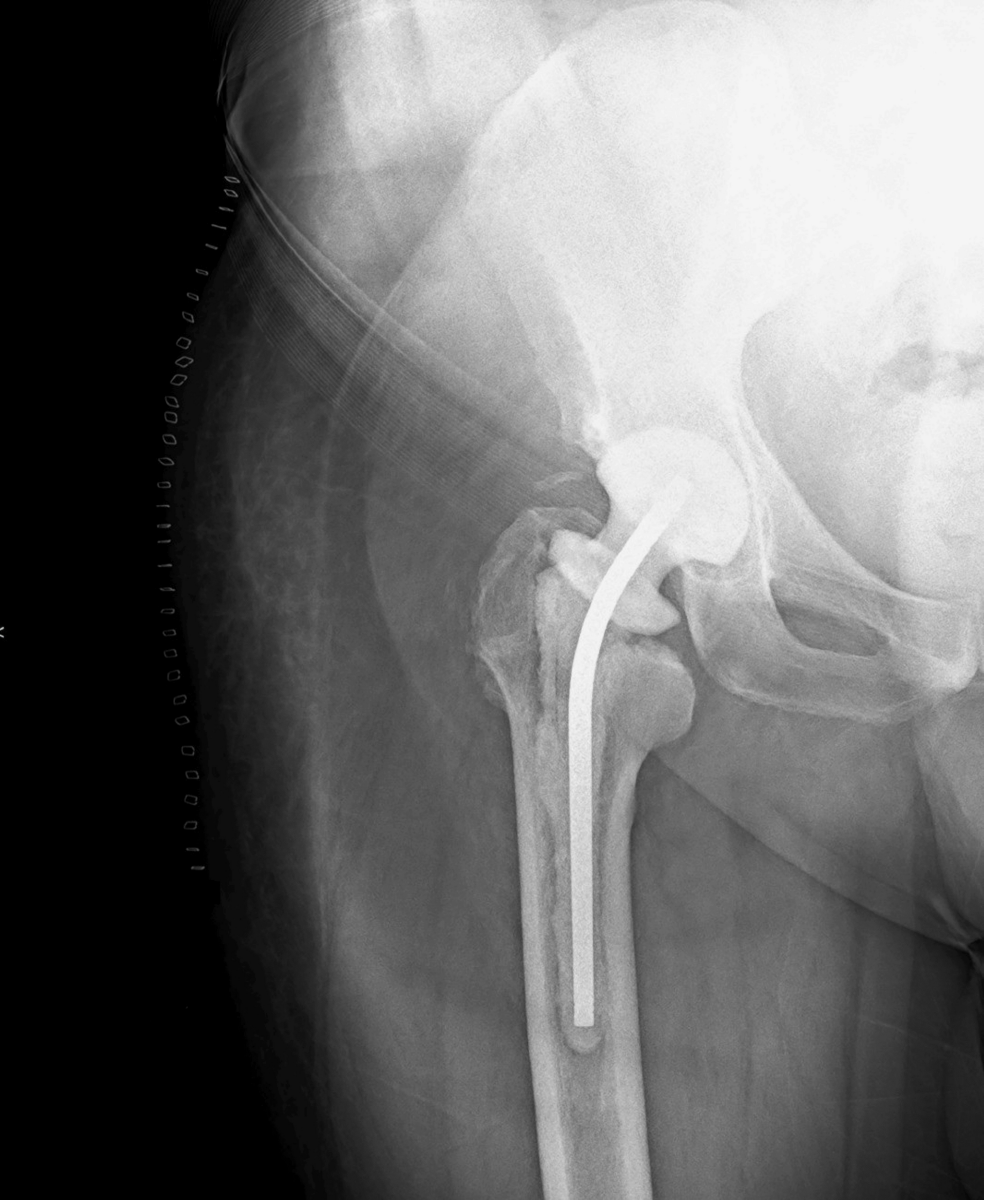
Radiograph of the antibiotic-loaded spacer inserted during the two-stage revision procedure.
